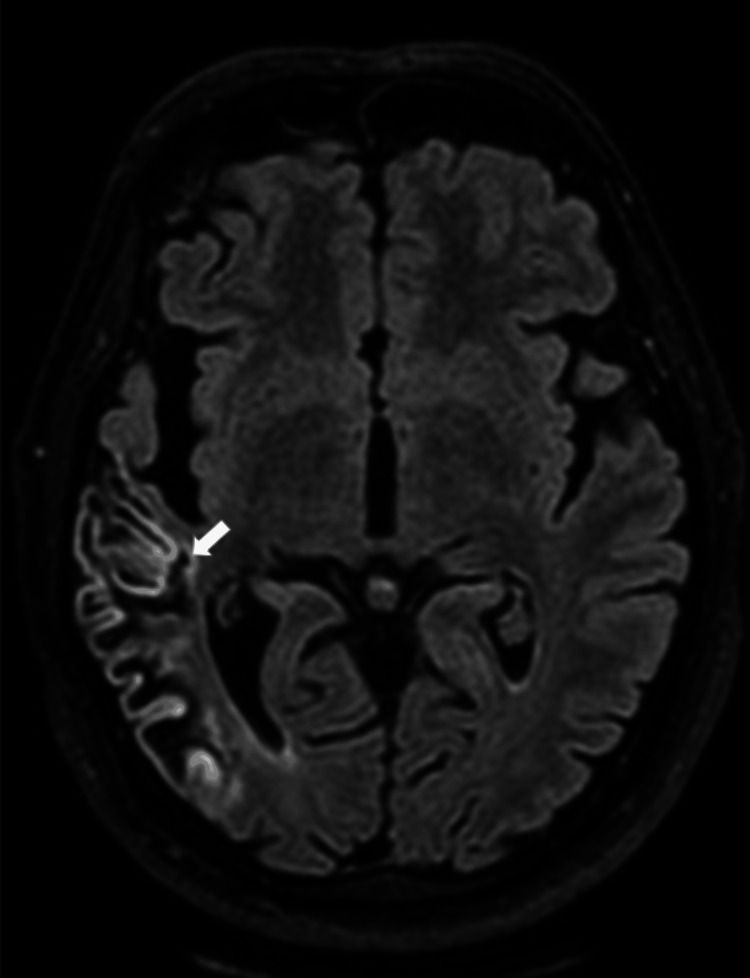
MRI brain FLAIR image (axial section) showing a hyperintense cortical lesion in the right temporal lobe FLAIR: fluid-attenuated inversion recovery; MRI: magnetic resonance imaging
